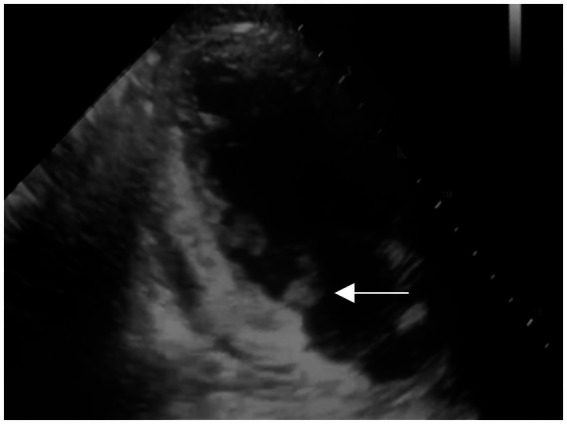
Transthoracic and contrast echocardiography of the patient 7 days after the first echocardiography (white arrow: mitral valve vegetation).
